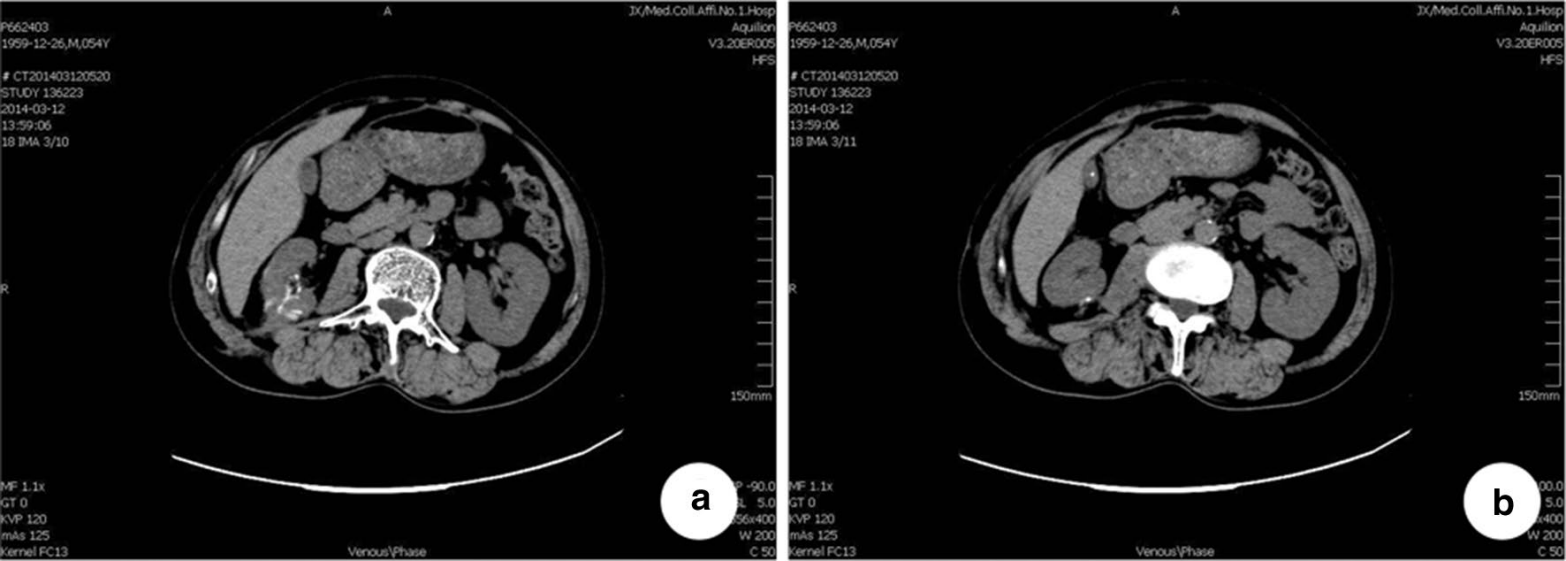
CT scan results of 4 months follow-up after surgery, a preoperative CT scan; b 4 month postoperative scan
